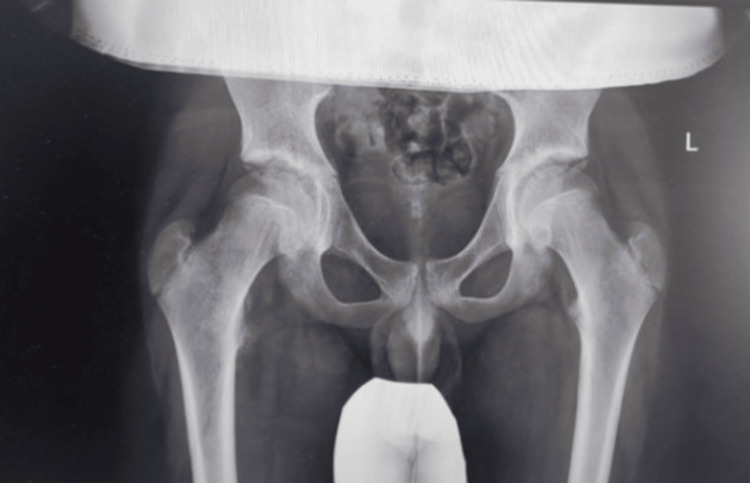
Pelvic X-ray three weeks after the injury
